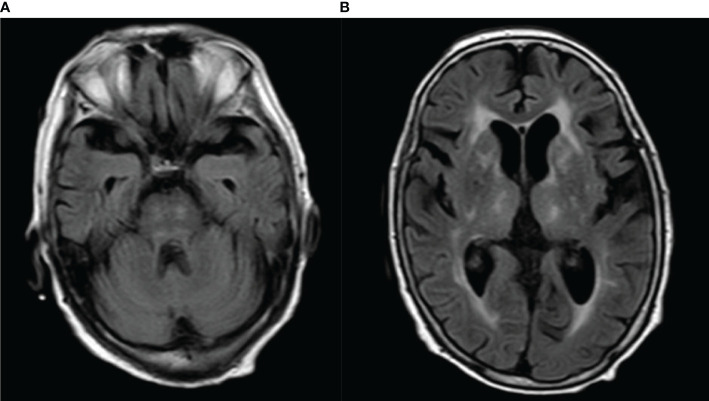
(A, B) FLAIR imaging revealed obvious high signal intensity in the pons and bilateral thalamus.
